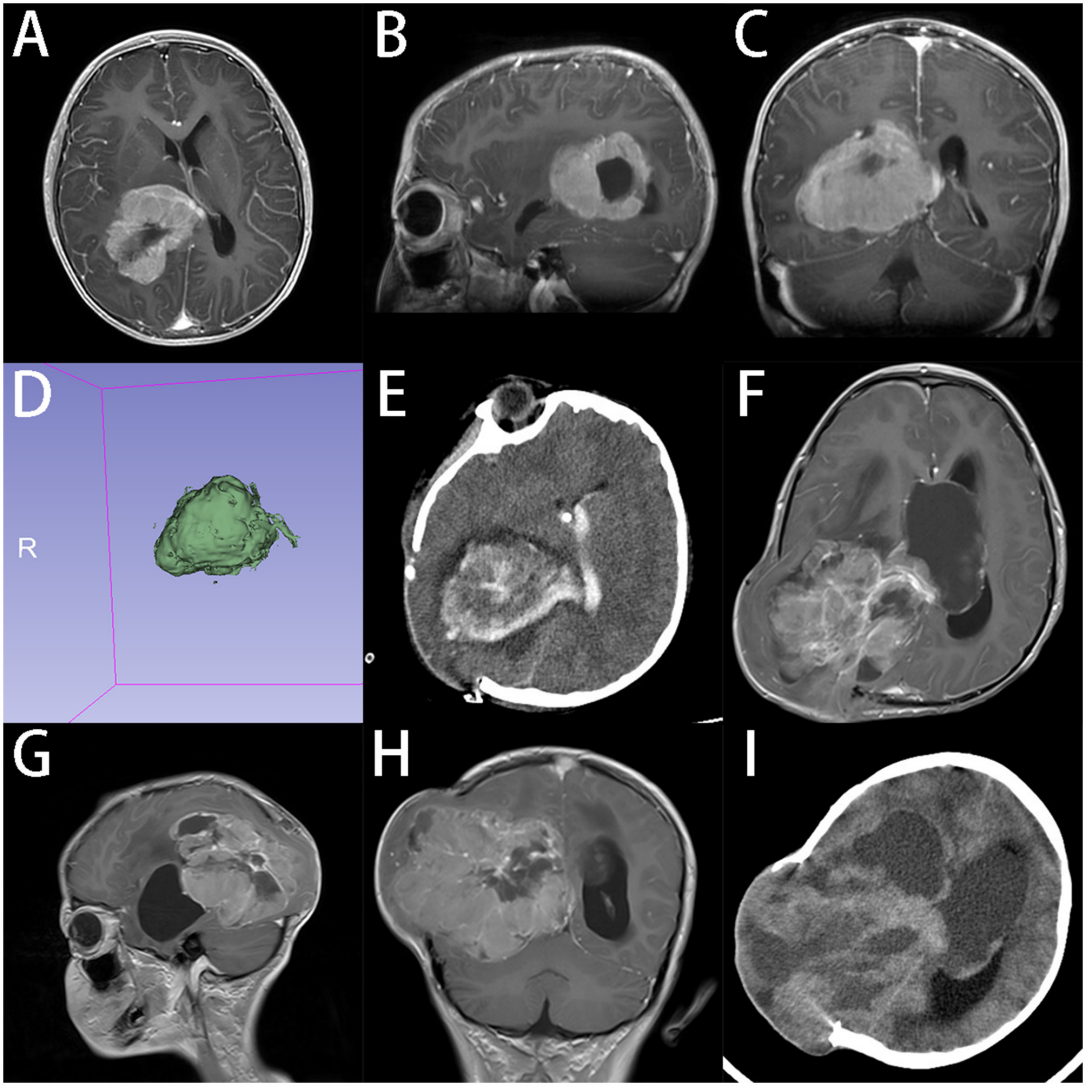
A 7-years-old boy with CPC in lateral ventricles. (A–C) Preoperative axial, sagittal and coronal gadolinium-enhanced T1-weighted MRI scan shows an inhomogeneous tumor with partial cystic changes in right lateral ventricle. (D) 3D model reconstruction of the tumor, the volume of tumor is 70.4 ml (E). CT shows that tumor was partially removed at 24 h after operation. Guardians reject the advice of secondary surgery after radiation and chemotherapy. (F–H) Axial, sagittal, and coronal enhanced T1-weighted MRI scan indicates that tumor recurred at 3 months after operation. (I) CT at 4 months after operation, tumor grew further and the condition of patient got worse. Patient died at 5 months after operation.
